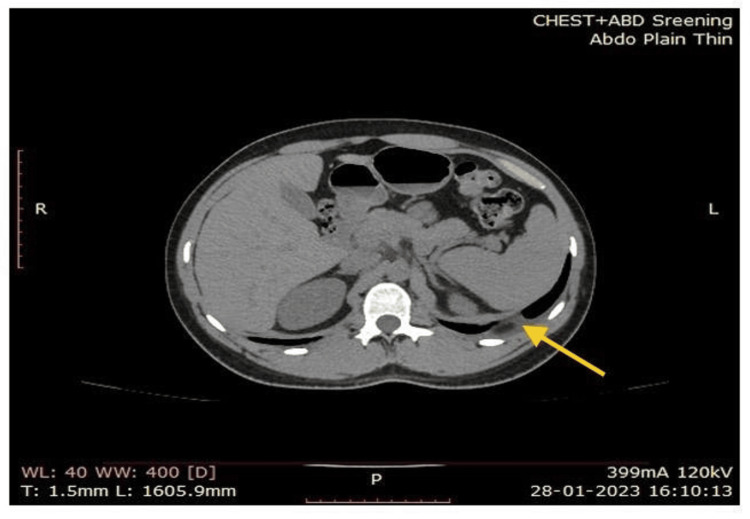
CT image showing minimal left pleural effusions
